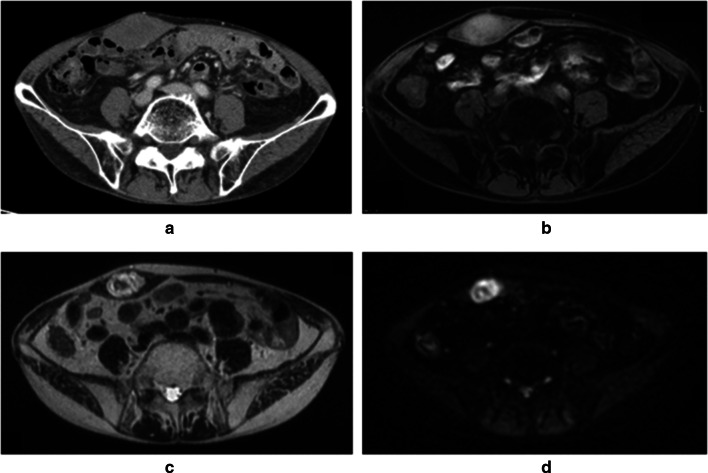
Abdominal CT scan reveals a tumor measuring 45 mm in a diameter at port site in right abdominal wall after 19 months later from the rectal cancer operation (a). Abdominal MRI, the mass was hypo-intense to muscle on T1 imaging (b), and hyper-intense on T2 (c). Some restricted diffusion was seen around the lesion (d)
